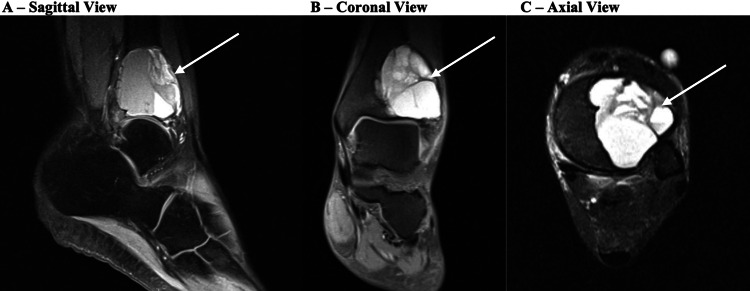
Prior MRI Sagittal (A), coronal (B), and axial (C) MRI views of the left ankle of the patient prior to her previous surgeries. The lesion is marked with arrows.
